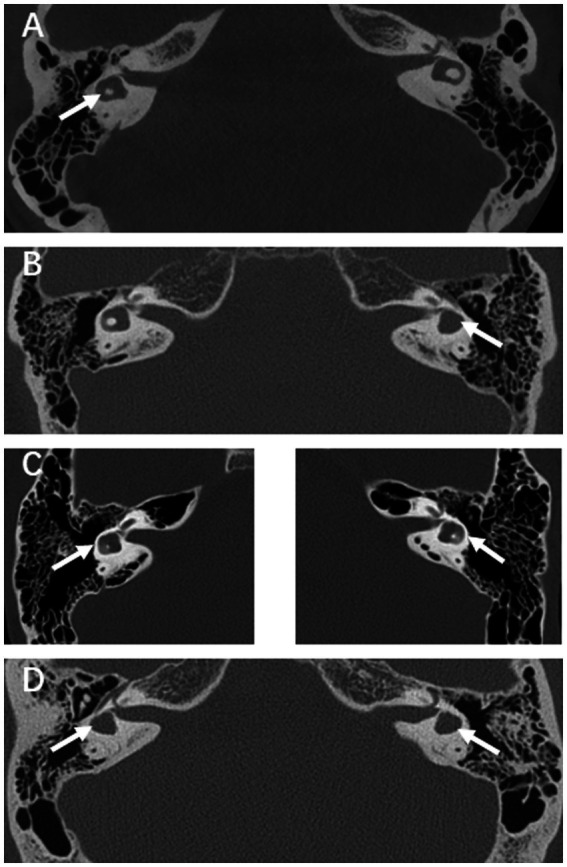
(A) HRCT scan of a patient with right LSCC malformation. (B) Example of a left LSCC malformation case with vestibular malformation. (C) Example of a bilateral LSCC malformations case, left and right side are placed separately. (D) Example of a bilateral LSCC malformations case with bilateral vestibular malformations.
